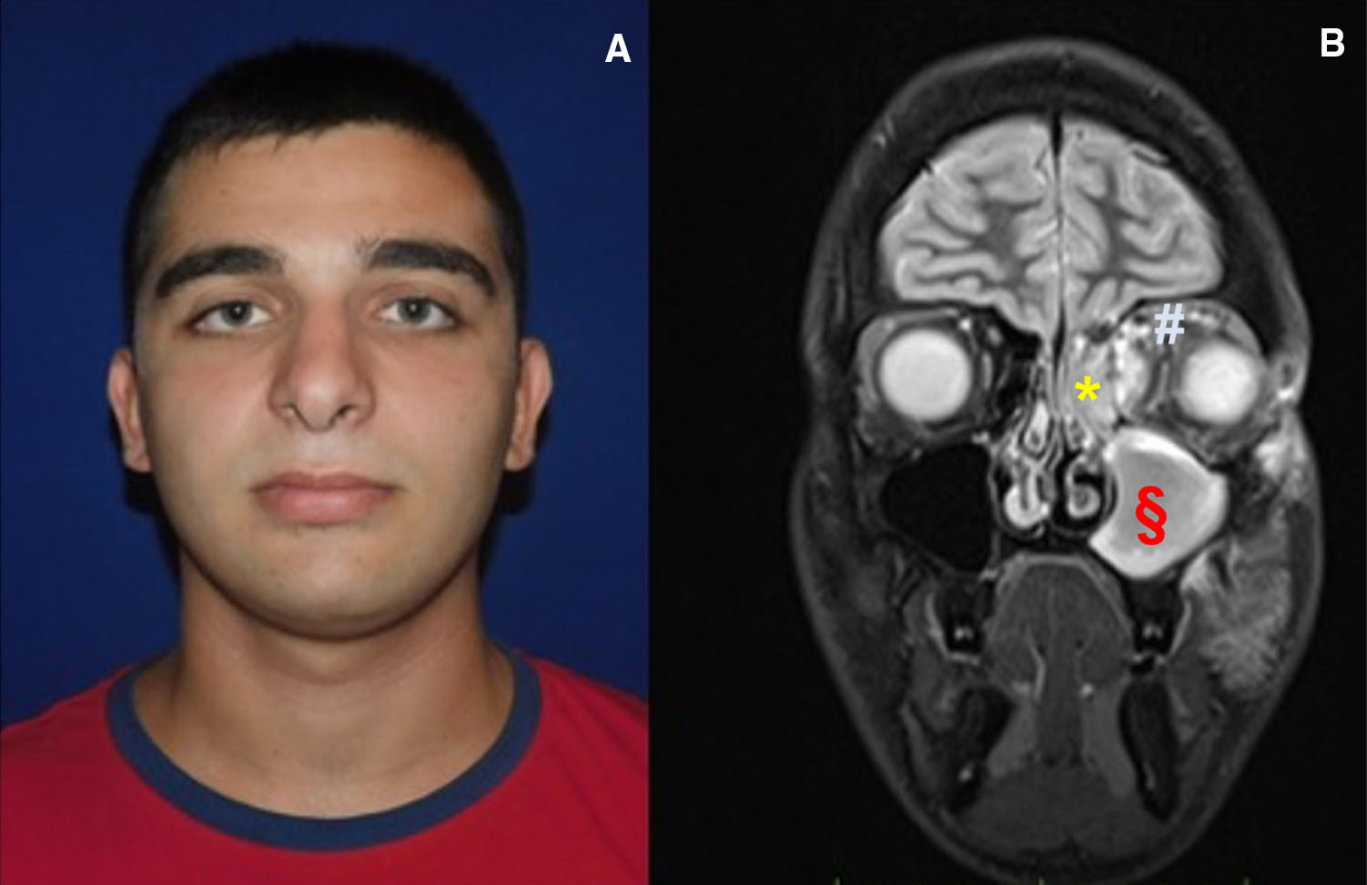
Case 2: Presurgical photo of patient in frontal section: swelling from abscess collection to supero-medial localization in the left orbit (a) and MRI Imaging in coronal section: maxillary sinus occupied by abscess (*), periorbital inflammatory degeneration of tissues (§), (b)
